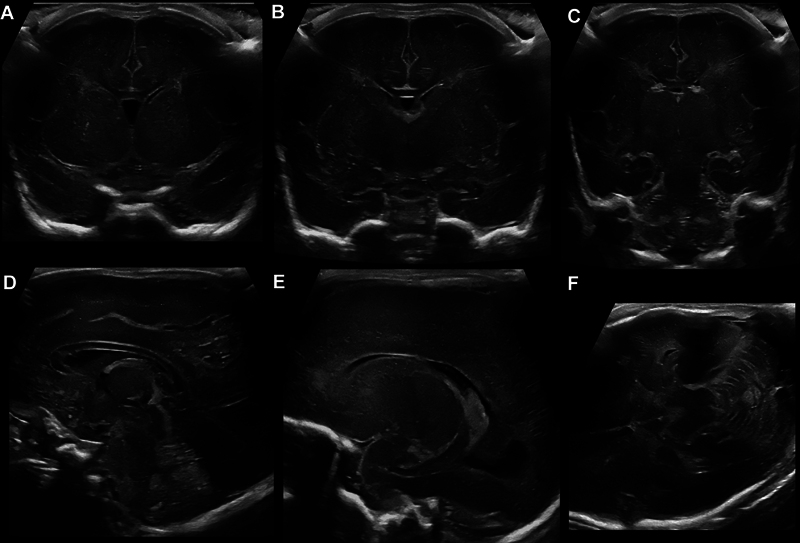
Normal cranial ultrasound images. Normal cranial ultrasound images of a former preterm infant at 35 weeks of gestation in coronal (
